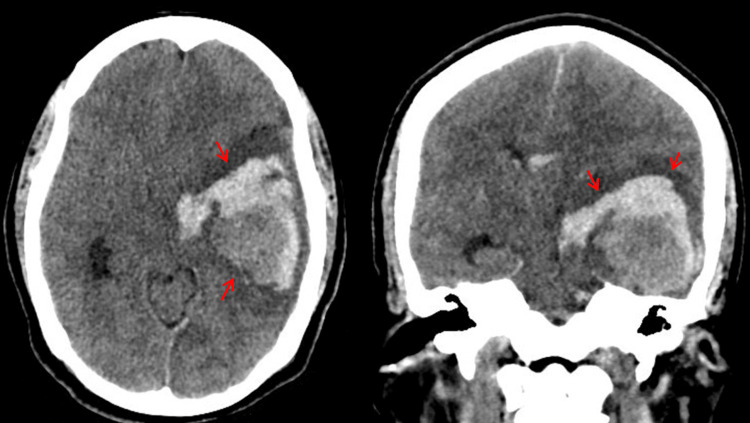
Non-contrast skull computed tomography showing the presence of a left fronto-temporo-parietal parenchymal hematoma Axial and coronal non-contrast brain computed tomography demonstrating a left fronto-temporo-parietal intraparenchymal hematoma measuring approximately 41 cc in volume, with heterogeneous hyperdensity, surrounding hypodense edema, and mild mass effect on the adjacent cortical structures (red arrows).
